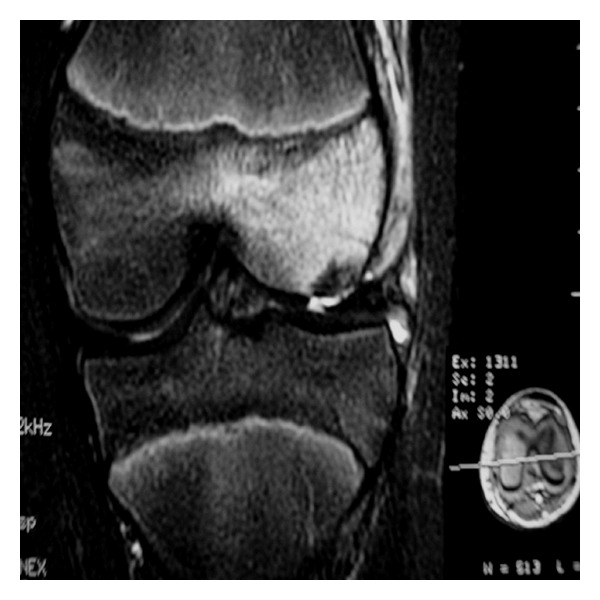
Preoperative MRI image of an osteochondral fracture of the lateral condyle in an 11-year-old girl.
